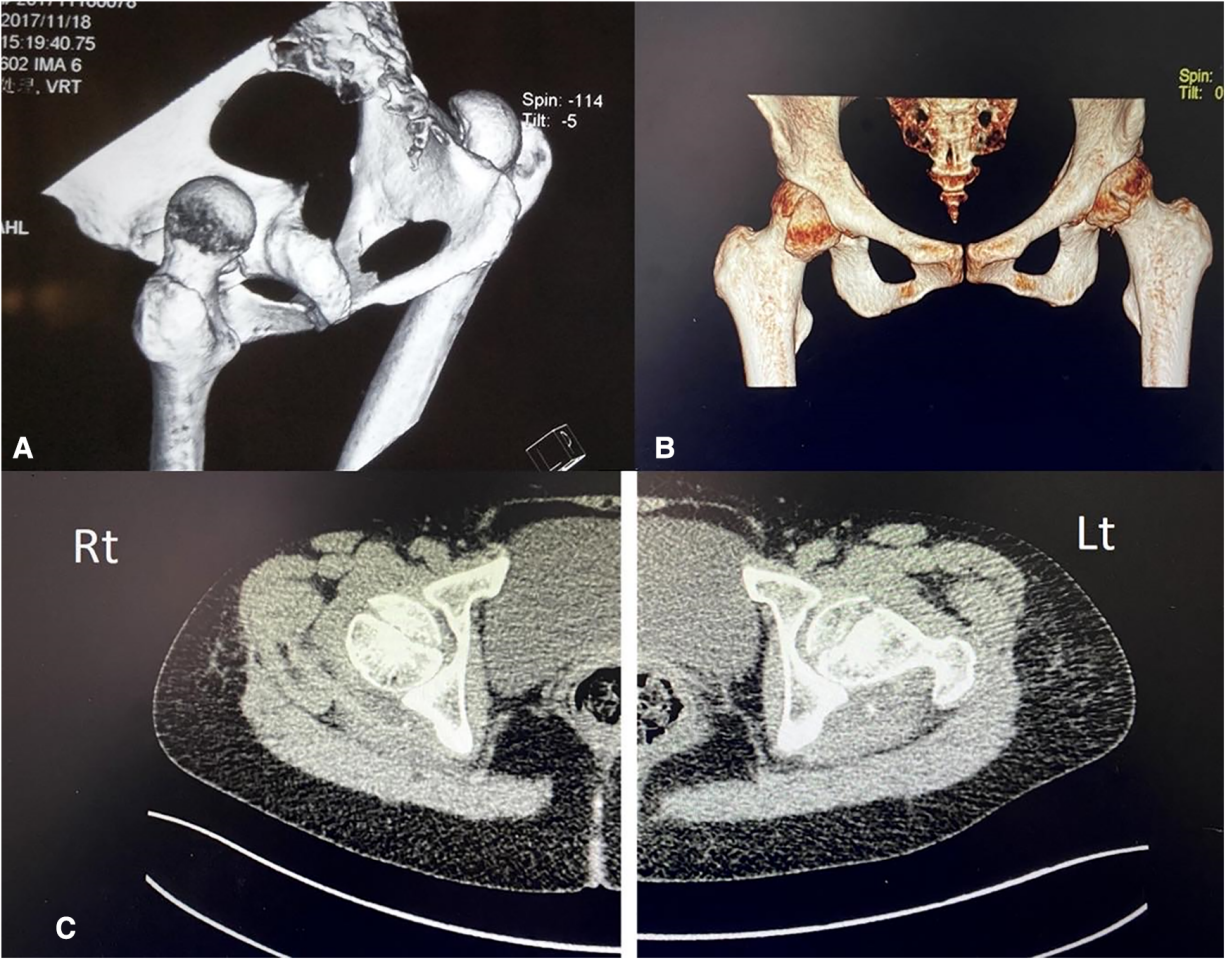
(A) 3D CT radiographs showing the bilateral posterior hip dislocation and femoral head fracture before the close reduction maneuver. (B) 3D CT radiographs showing bilateral relocated hip after reduction along with inferior part of femoral head fractures. (C) Plain CT transverse section of images showing bilateral intra-articular fractures of the femoral head.
